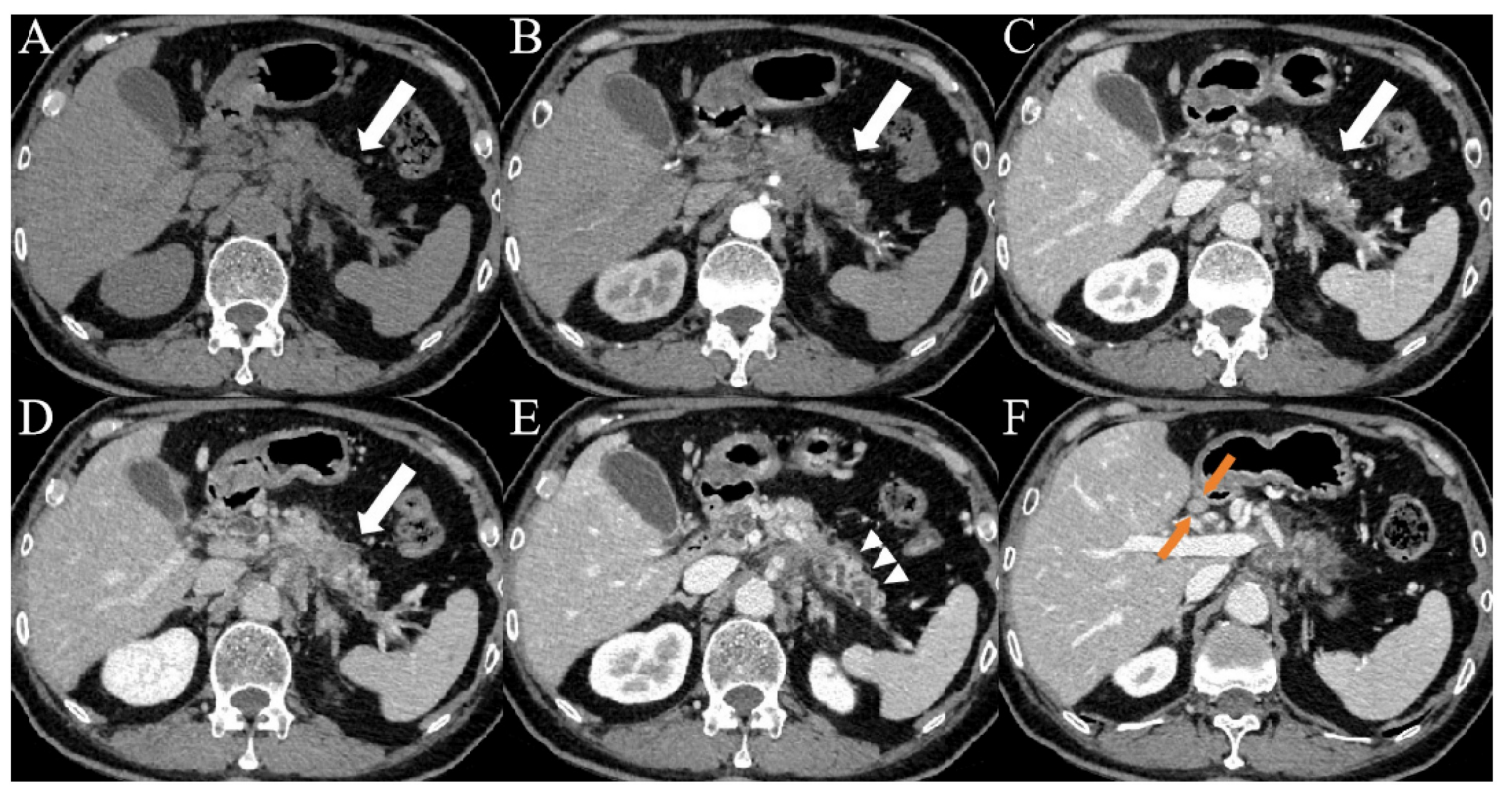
Images from a patient with PDAC. (A-D) CT images showed patchy areas of low density within the body of the pancreas (white arrows) with a hypovascular pattern, the margin of which was ill-defined. (E) Contrast-enhanced CT images during the portal venous phase showed duct dilatation and marked tail atrophy (arrowheads). (F) CT imaging showed a significantly enlarged lymph node (orange arrows) in the hepatogastric space, with a short axis measuring approximately 1.1 cm. The patient's CA19-9 level was 78.3 U/mL (> 37 U/mL), and the D-dimer level was 14.7 mg/L (> 0.84 mg/L).
